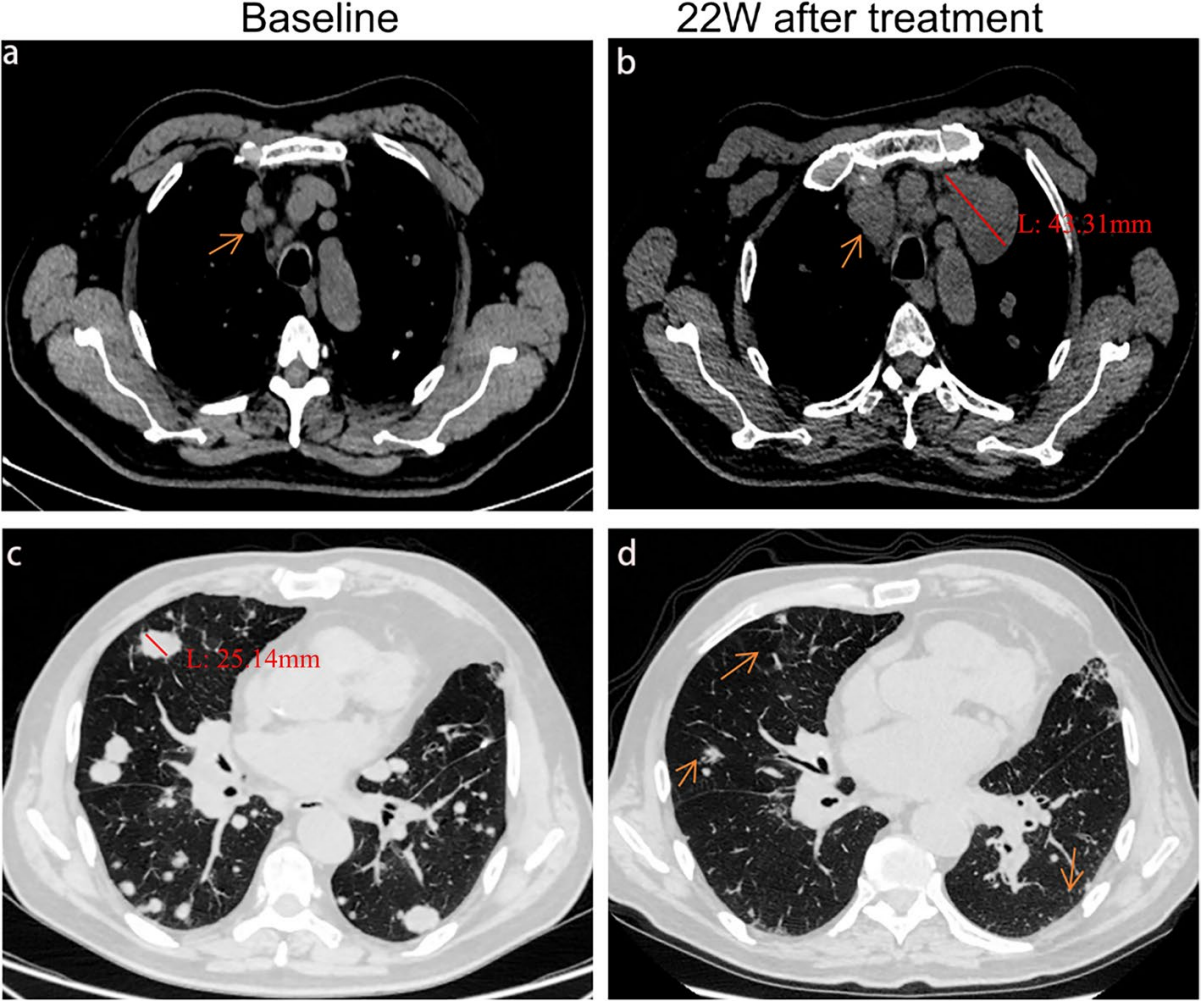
Representative radiological data of patients with dissociated responses. A 67-year-old man with advanced lung adenocarcinoma. He started PD-1 inhibitors combined with radiotherapy as a fifth-line therapy. The radiation site was a metastatic lesion of the right iliac crest. After 22 weeks of treatment, his pulmonary metastasis lesions (the largest: 25 mm) reduced in size (c, d), although two new metastatic lymph nodes (the larger: 43 mm) in the mediastinum were detected on the CT scan (a, b); therefore, DR was diagnosed
